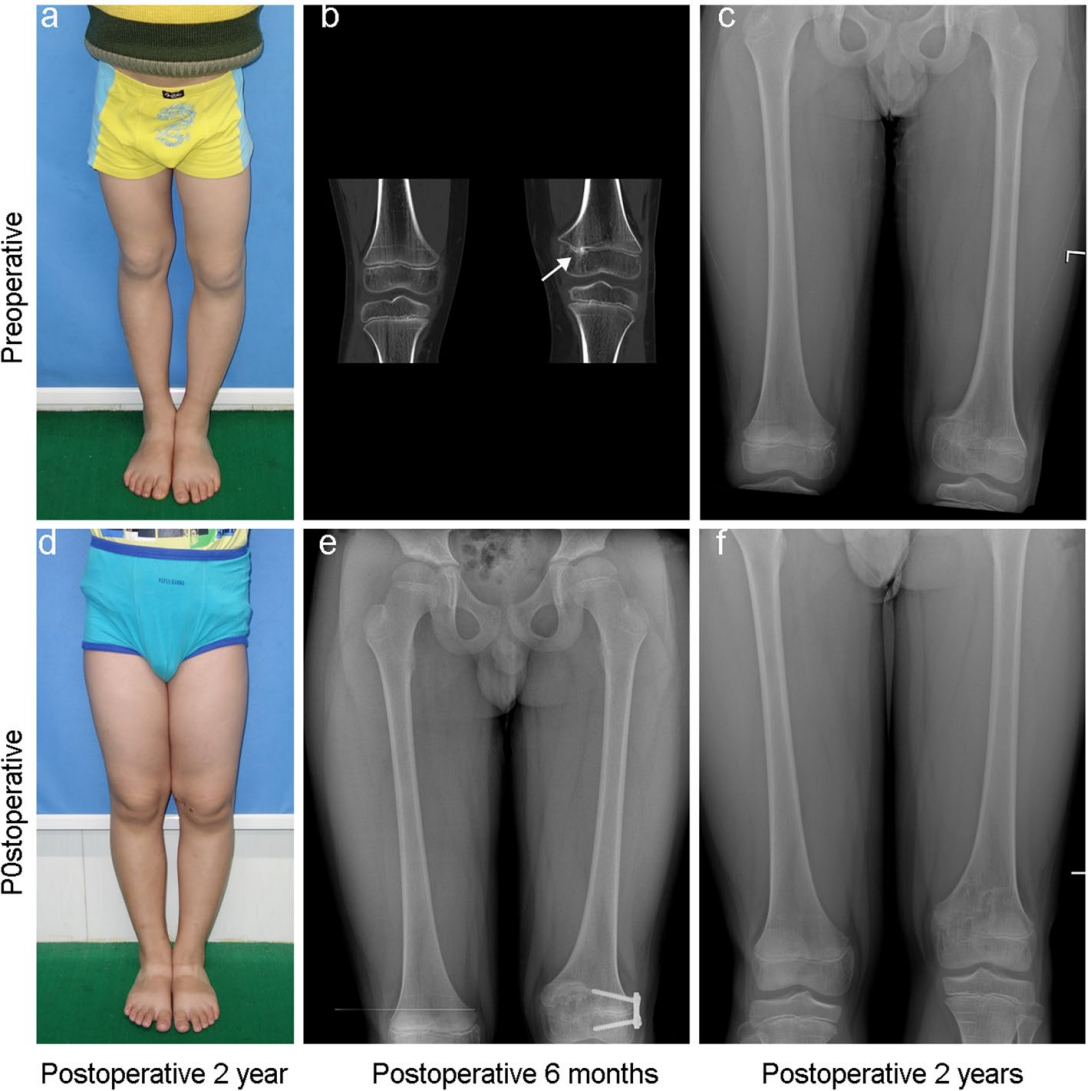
Typical case of bar resection (Case 10). The gross view (a), coronary CT (b), and anterior-posterior radiograph (c) of 9 years and 6 months old boy before surgery; (d) The gross view of the patient at postoperative two years; (e) The anterior-posterior radiograph of the patient at postoperative 6 months; (f) The anterior-posterior radiograph of the patient at two years
